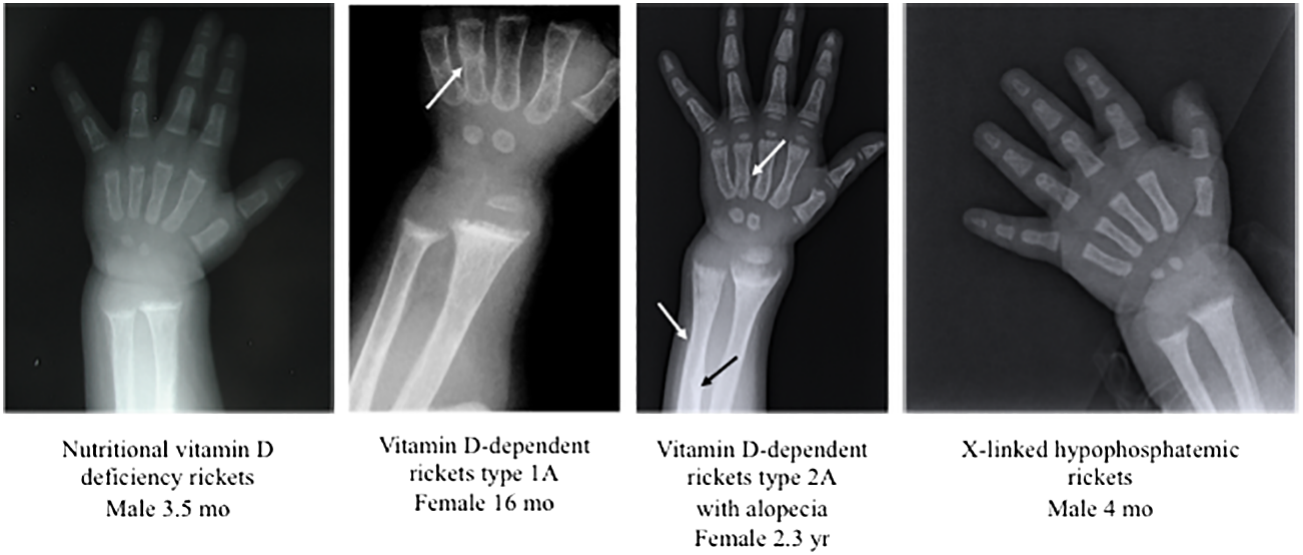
Radiographic features at the wrist and at the hand in patients with nutritional vitamin D deficiency rickets and some forms of hereditary rickets. In patients with hereditary rickets, the diagnosis was confirmed by genetic analyses. All patients showed widening and fraying of the epiphyseal plate and metaphyseal concavity of the ulna. The white and black arrows showed fractures.
